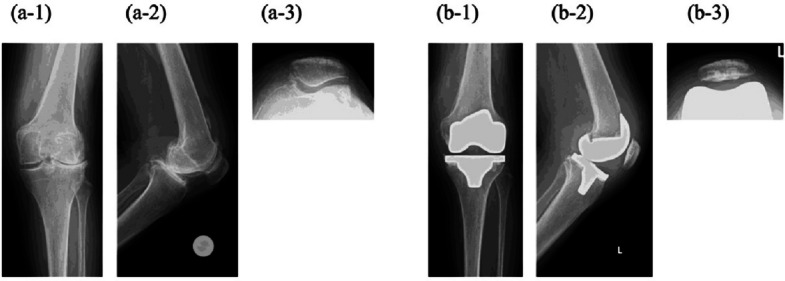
Radiographic findings in a 76-year-old woman. (a) pre-operative images: (1) anteroposterior, (2) lateral, and (3) skyline views. The KL classification was grade IV, the Ranawat classification was type II, FTA was 165°, and the HKA angle was valgus 11°. (b) post-operative image: (1) anteroposterior, (2) lateral, and (3) skyline views. The FTA and HKA angles improved to 175° and 0°, respectively. Implant positioning was accurate with an α angle of 96°, β angle of 89°, γ angle of 8°, and δ angle of 87°. The patellar position was appropriate on the skyline view. RTKA: robotic arm-assisted total knee arthroplasty, KL: Kellgren–Lawrence classification, FTA: lateral femorotibial angle, HKA: hip–knee–ankle angle
